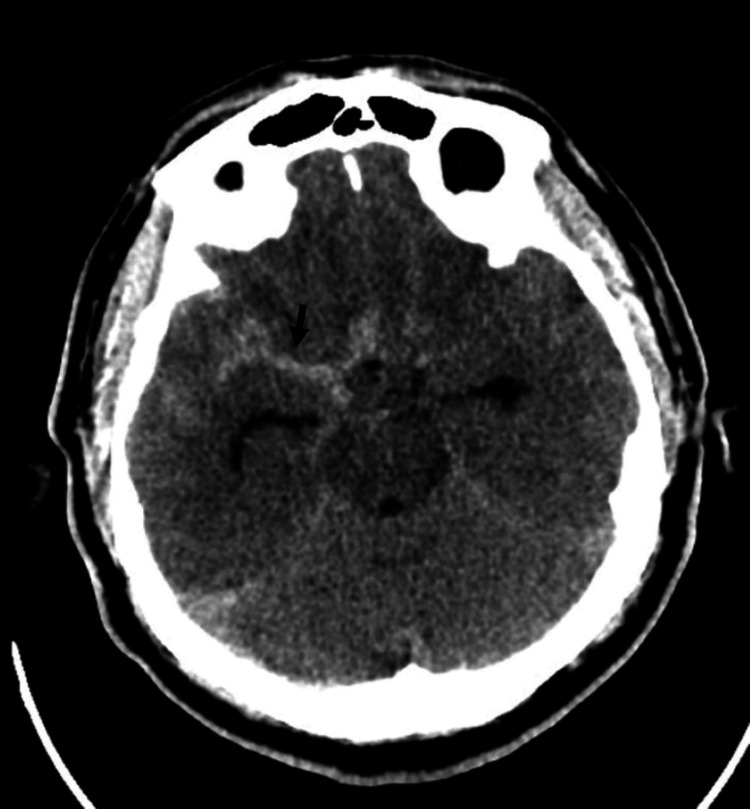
Computed tomography of brain without contrast (axial view) The image shows subarachnoid hemorrhage (black arrow) within the basal cisterns, more pronounced on the right side, right sylvian cistern, as well as the interhemispheric fissure and parasagittal sulci.
